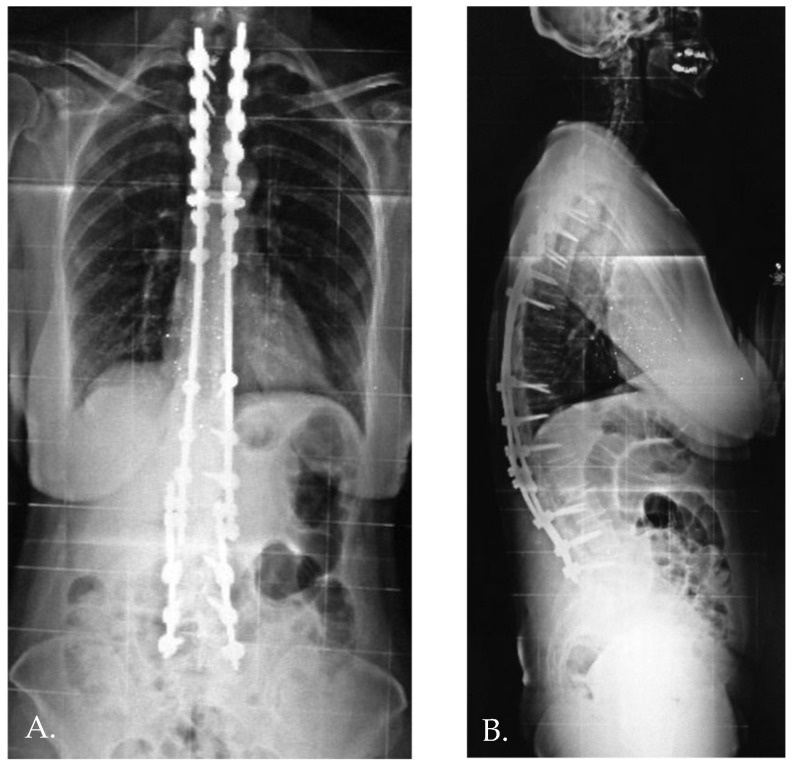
Anteroposterior and standing lateral radiographs (A,B) after revision surgery.
